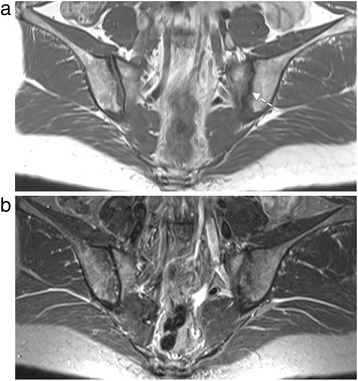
Erosion of the left iliac bone on T1 weighted spin echo magnetic resonance imaging (MRI) (a) and in the absence of inflammation on short tau inversion recovery MRI (b)
